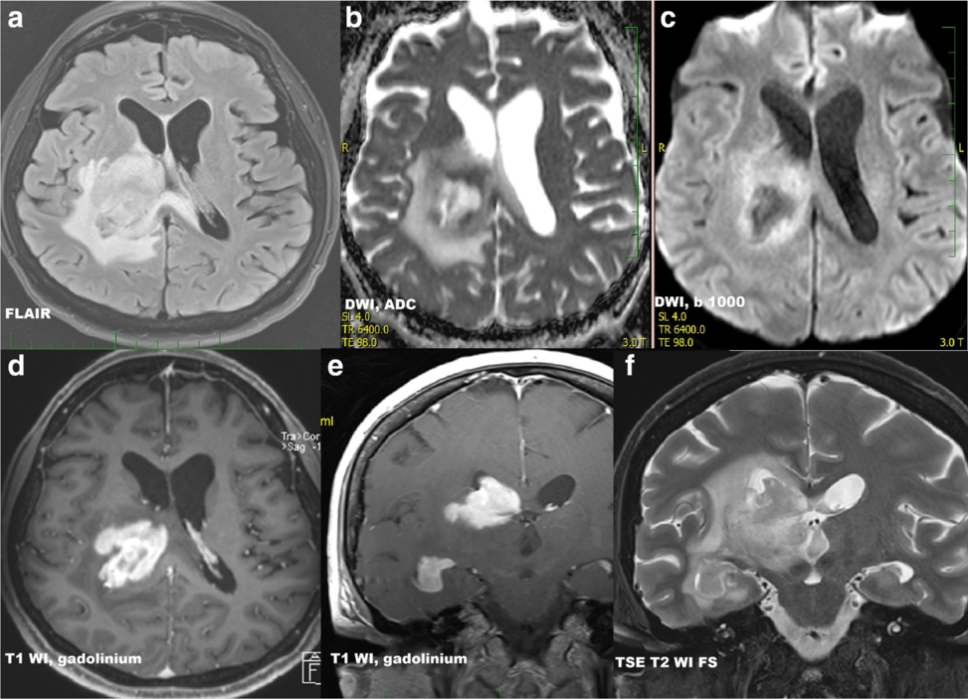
a) FLAIR, axial scan b) DWI, ADC map, axial scan c) DWI, b=1000, axial scan d) SE T1 WI after intravenous gadolinium contrast administration, axial scan e) SE T1 WI after intravenous gadolinium contrast administration, coronal scan f) FSE T2 WI with fat saturation, coronal scan
